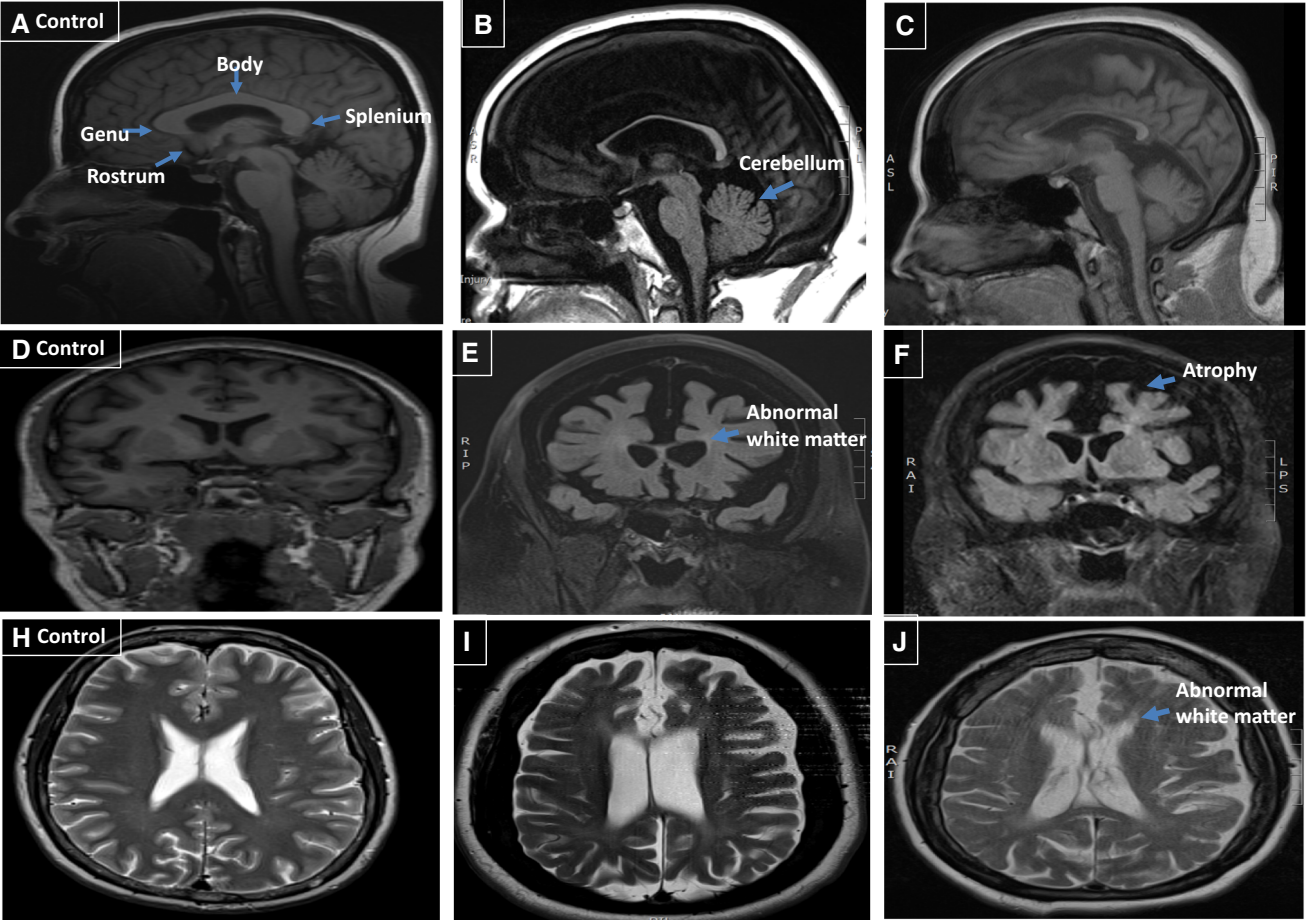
Sagittal MRI sequence; a control MRI with corpus callosum labeling. b Proband II:1 and c case II:2 showing thinning of the corpus callosum and cerebral atrophy. Coronal MRI sequence; d control. e Proband II:1 and f II:2 showing generalized atrophy with periventicular white matter abnormalities. Axial MRI sequence; h control. i Proband II:1 and j II:2 showing periventicular white matter abnormalities
